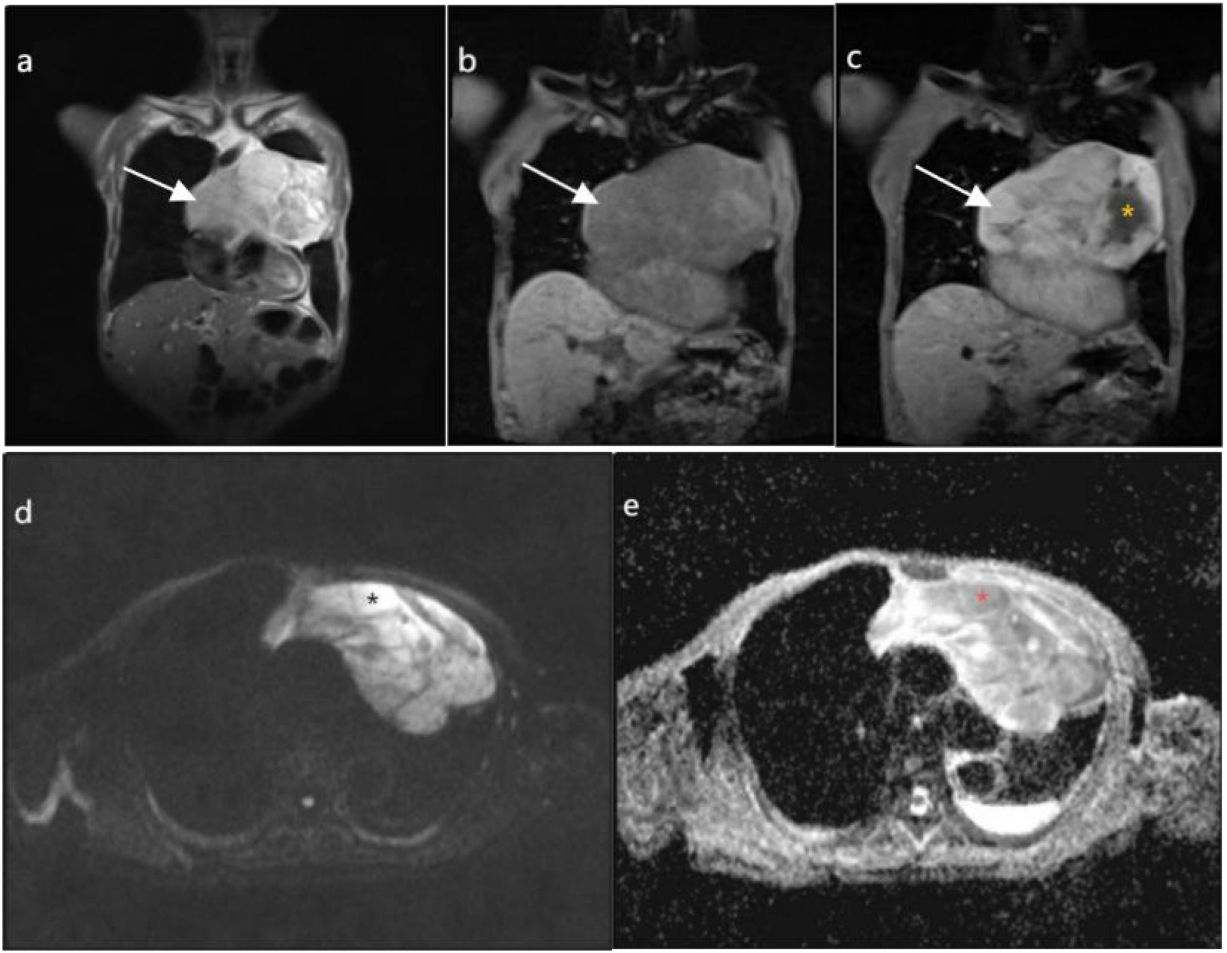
(A, B) depict coronal T2-weighted imaging (T2WI) and T1-weighted imaging (T1WI), respectively, demonstrating an irregular mass in the anterior mediastinum (highlighted by white arrows), displaying mixed T2 and T1 signal intensities with internal low signal septations on T2WI, measuring approximately 167mm×97mm×106mm with well-defined margins. (C), on coronal T1WI post-contrast imaging, reveals heterogeneous enhancement with irregular cystic necrotic areas within (denoted by yellow asterisks). (D) shows diffusion-weighted imaging (DWI) with a b value of 800, demonstrating diffusely high signal intensity in the solid component (denoted by black asterisks). (E) displays an apparent diffusion coefficient (ADC) value of 1.430×10-3mm2/s (denoted by red asterisks).
